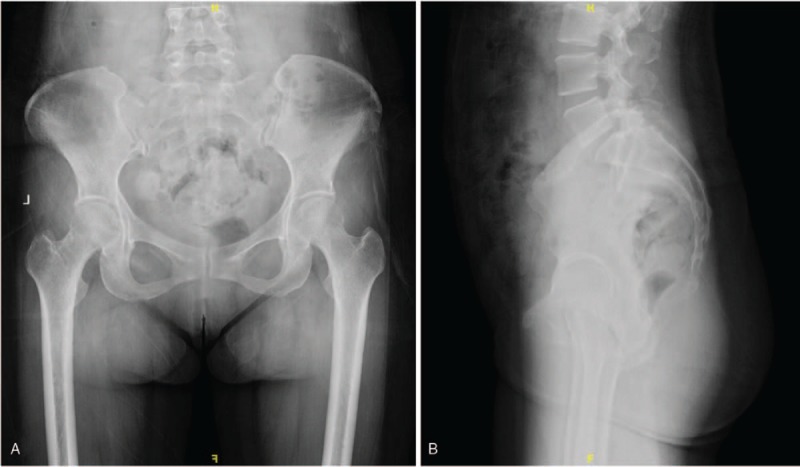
(A, B) Preoperative X-rays revealing sacral lesions with high suspicion of soft tissue tumors.
